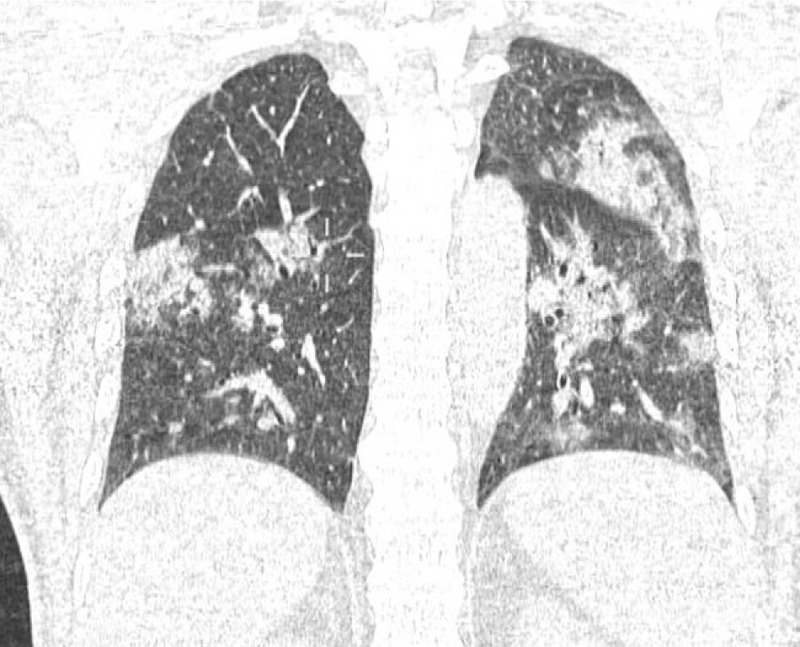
Chest CT-scan in coronal cut showing ground-glass opacities suggestive of pulmonary infection by the novel coronavirus.
